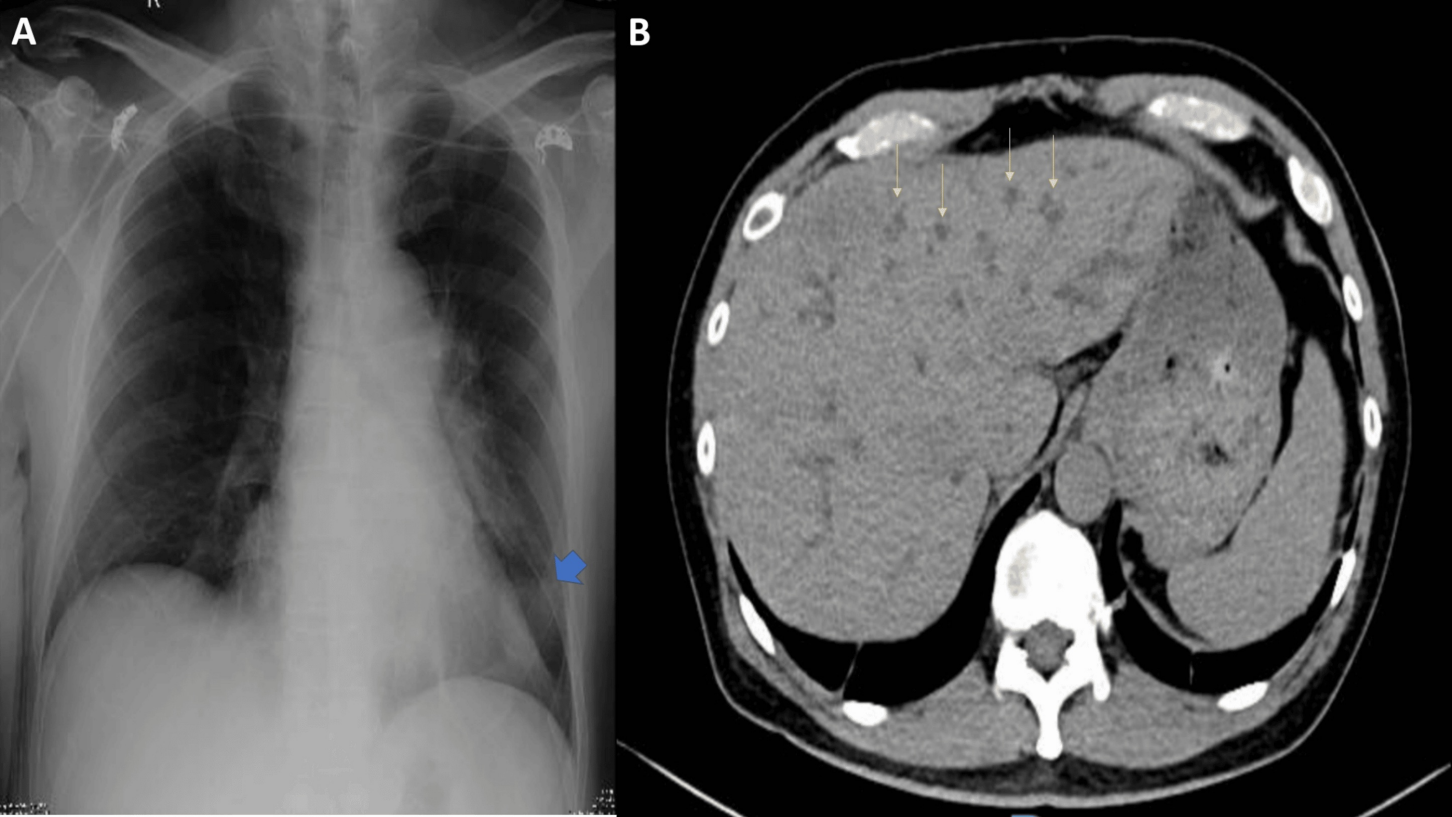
(A) Chest X-ray at admission showing a left lower lobe mass. (B) Dynamic liver CT scan demonstrating multiple nodules in the liver parenchyma. Blue arrow: left lower lobe lung mass. Yellow arrows: hepatic nodules.
